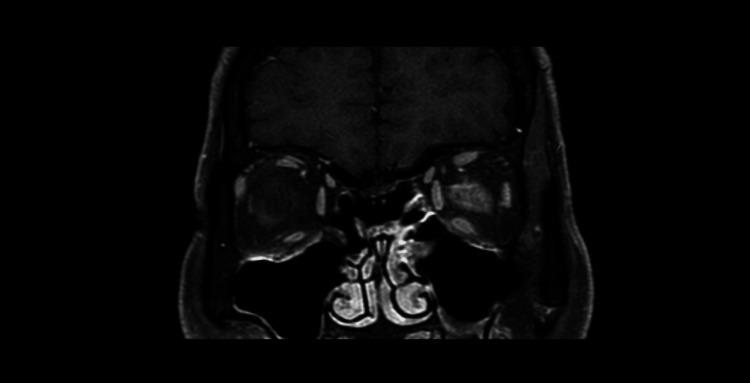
Coronal T2-weighted magnetic resonance imaging (MRI) showing structural changes in the left optic nerve, with no visible changes in the brain parenchyma.
